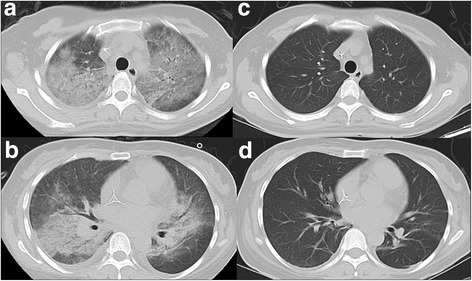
a, b Helical computed tomographic scanning showing bilateral diffuse ground glass opacities, partialpleura thickening, crescent shaped liquid density of bilateral chest wall. c, d A repeat CT scan 7 days after glucocorticoid and antibiotic therapy showing almost complete resolution of the interstitial infiltration
